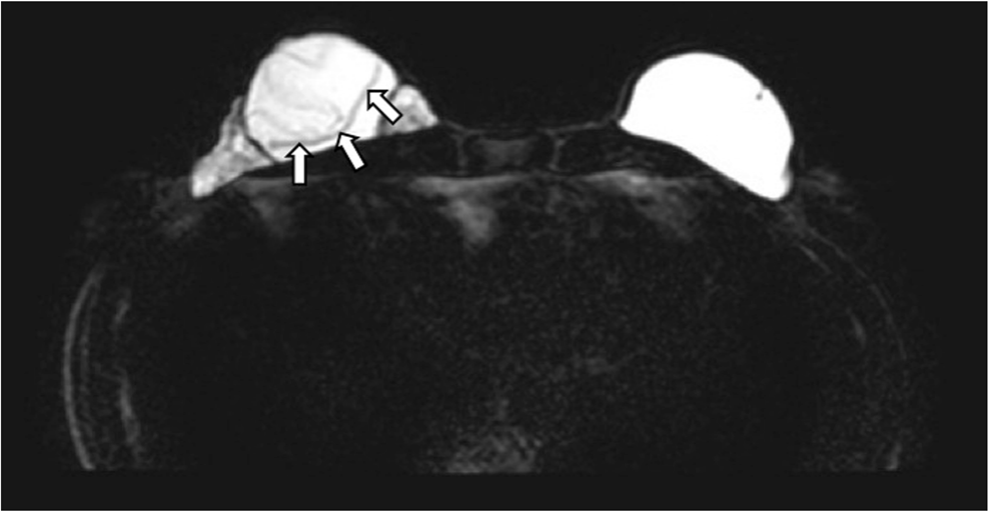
Subcapsular line sign. Silicone-only axial MR image shows a hypointense line (arrow) running almost parallel to and just beneath the fibrous capsule in right breast, due to a thin layer of silicone between the shell and the fibrous capsule“Pull away”, “open loop”, or “undercapsular streaks” sign. This refers to the presence of hypointense lines, which are characteristically parallel to the capsule, due to localized leakage of implant material. This causes a small displacement of the capsule [2].“Keyhole”, “noose”, or “inverted-loop” sign. It appears when there is further progression of the “pull away sign”. It is the leakage of implant material into the invaginated implant shell, with the two membranes not touching each other [2, 3] (Fig. 10).
